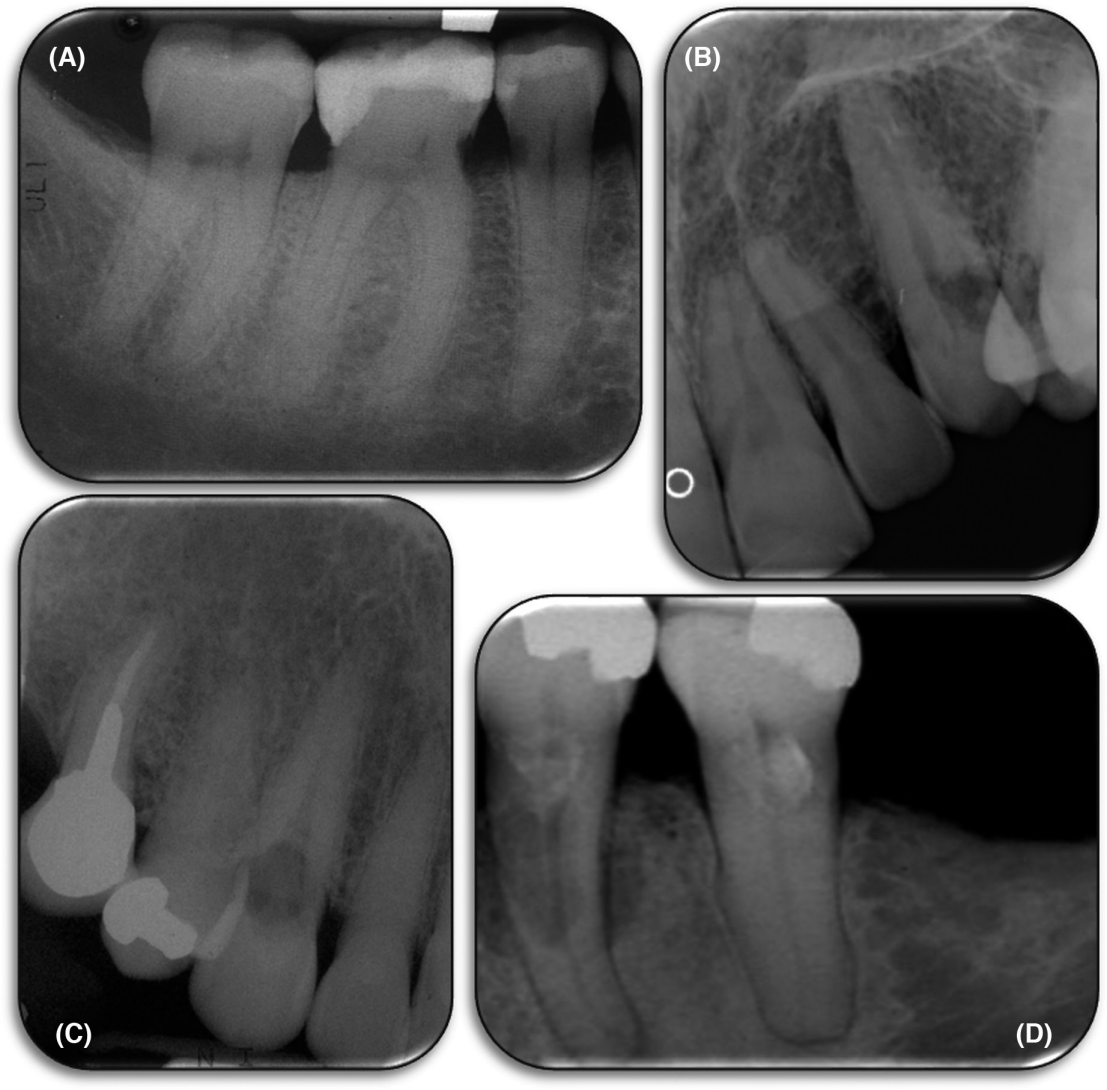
External Invasive Resorption. Periapical radiographs showing examples of the four stages of this type of resorption, according to Heithersay (1999b). (A) Class 1—mesial surface of tooth 36 and distal surface of tooth 35; (B) Class 2—distal and labial surfaces of tooth 23; (C) Class 3—palatal surface of tooth 13; (D) Class 4 mesial, buccal and lingual surfaces of tooth 34
